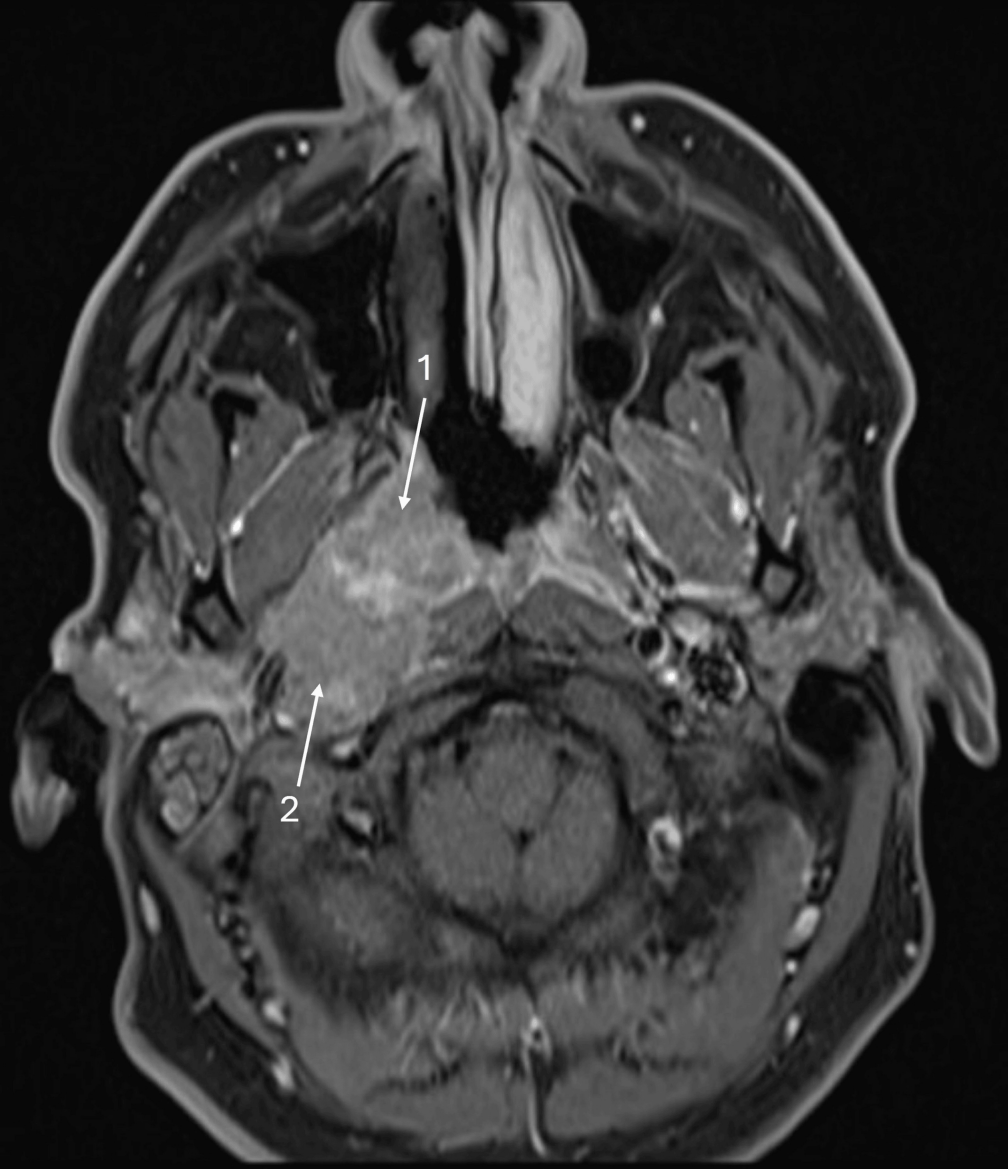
MRI internal acoustic meatus MRI internal acoustic meatus showing a right nasopharyngeal mass (1) extending into the right parapharyngeal space (2).
